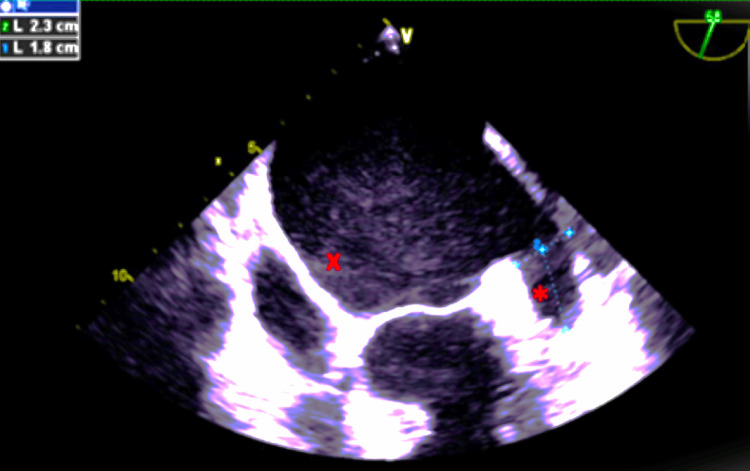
Pre-LAAC transesophageal echocardiogram at 68 degrees. * = left atrial appendage; X = left atrium; LAAC = Left Atrial Appendage Closure
